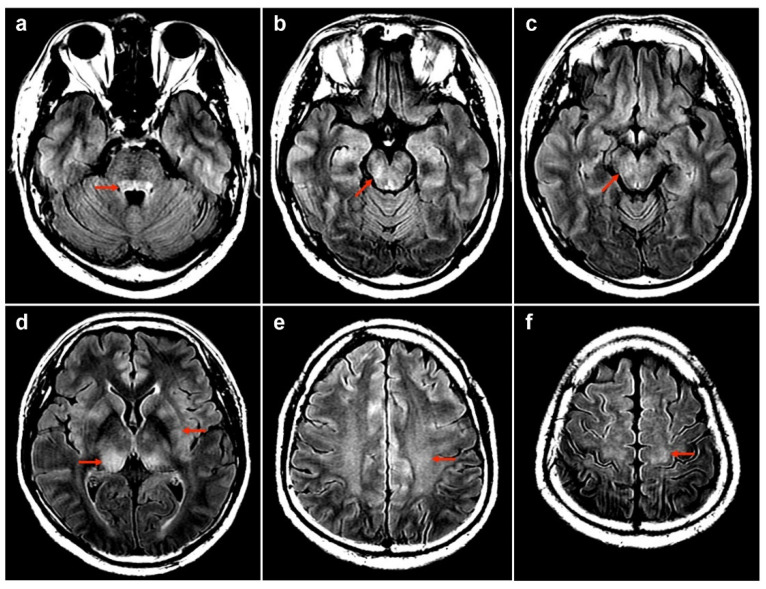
Brain MRI: Bilateral poorly demarcated T2 FLAIR hyperintensities at brainstem (a–c), pulvinar thalami (d), putamen (d), centrum semiovale (e), and subcortical white matters (f) are marked by red arrows in the image.
